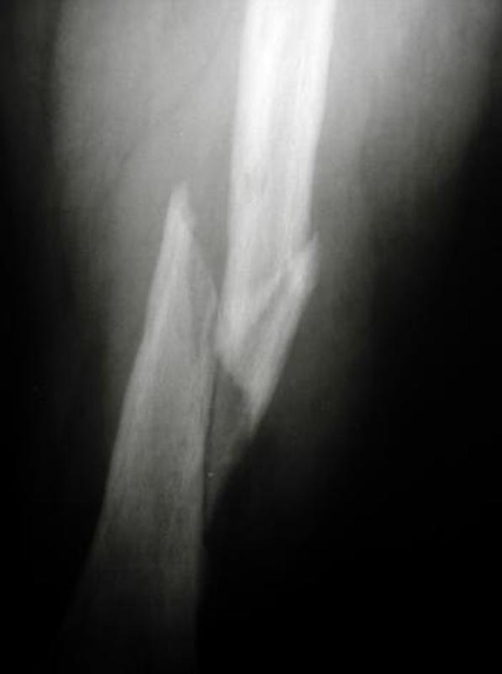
Radiograph at presentation showing the femoral diaphyseal fracture.
